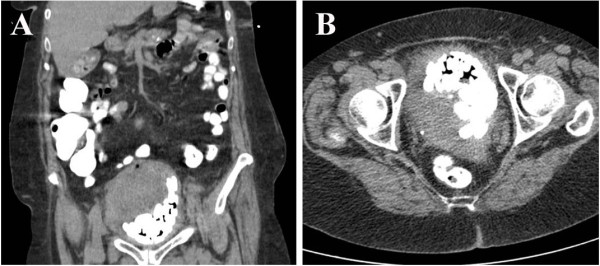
CT of the abdomen/pelvis with oral contrast, coronal view (A) and axial view (B).
